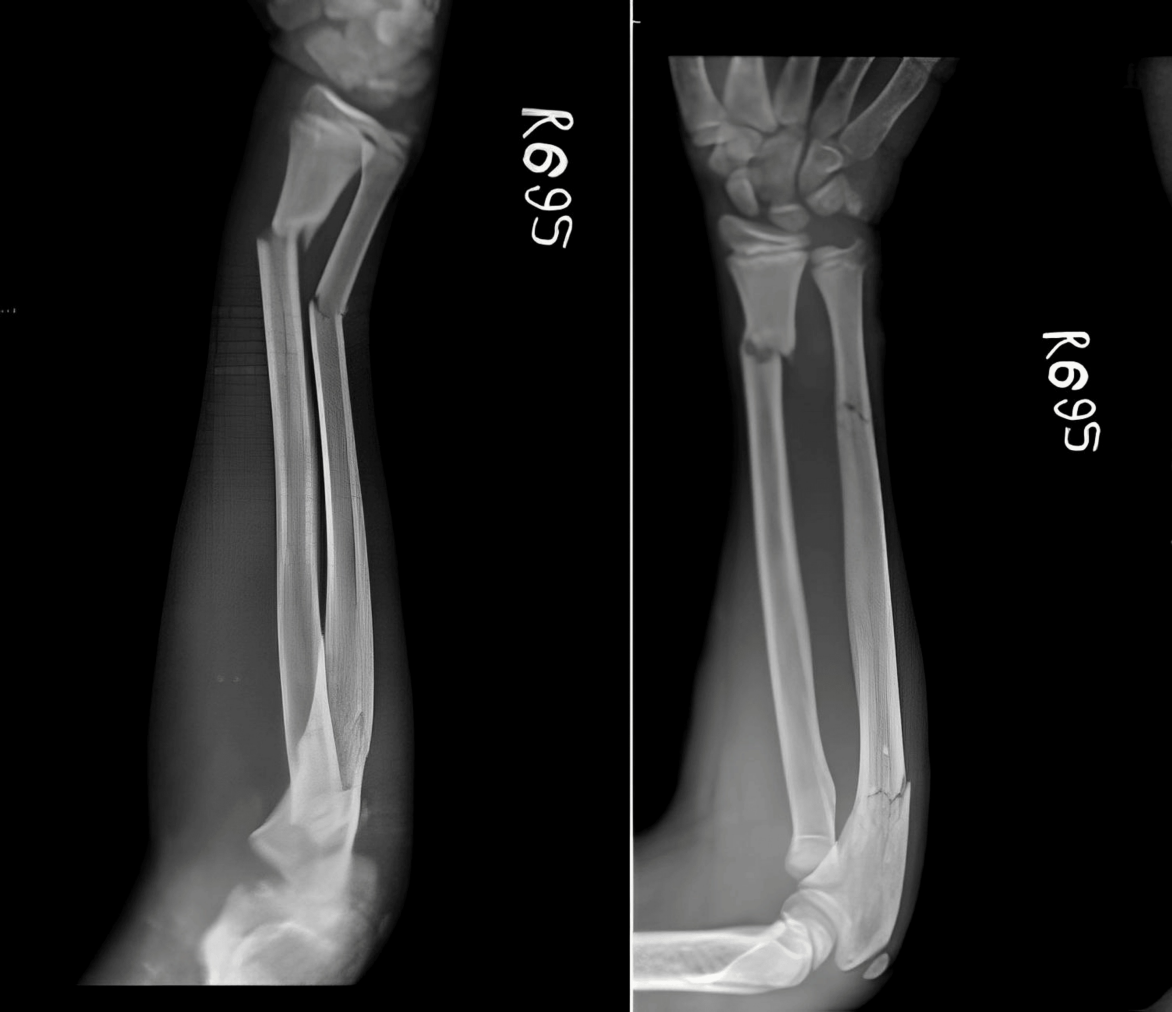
Preoperative X-ray of case 1 showing distal fracture of the radius with segmental ulna fracture and radial head fracture
